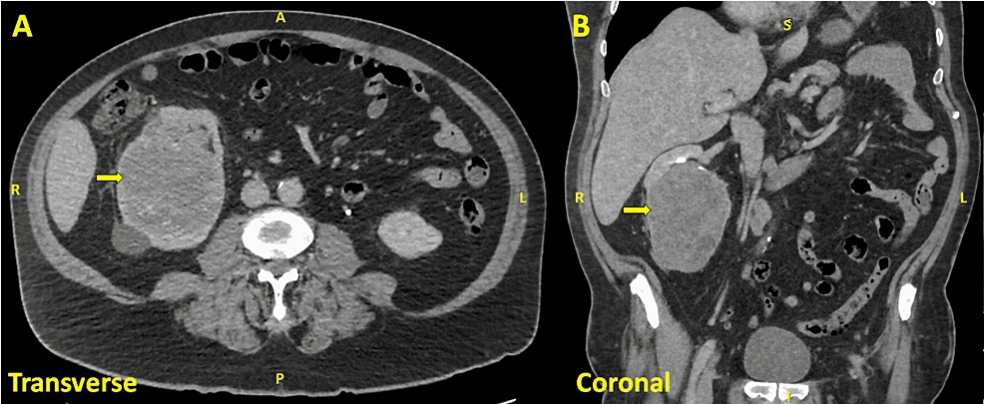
CT Urogram There is an enhancing mass in the lower pole of the right kidney, potentially arising from the renal pelvis, in transverse (A) and coronal (B) planes measuring 9.3 x 10.8 x 11.3 cm (arrows). There are linear areas of hyperdensity within the mass that could reflect calcifications or hemorrhage. The lesion appears to be arising from the renal pelvis. Enlarged retroperitoneal lymph nodes are present in the portacaval region.
